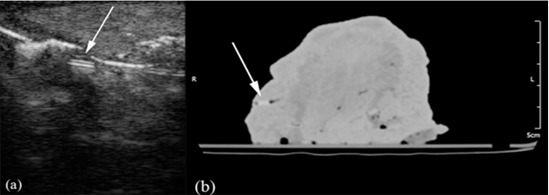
a: Arrow points to a plastic foreign body on the ultrasound image. b: Arrow indicates a plastic foreign body on the CT image.
